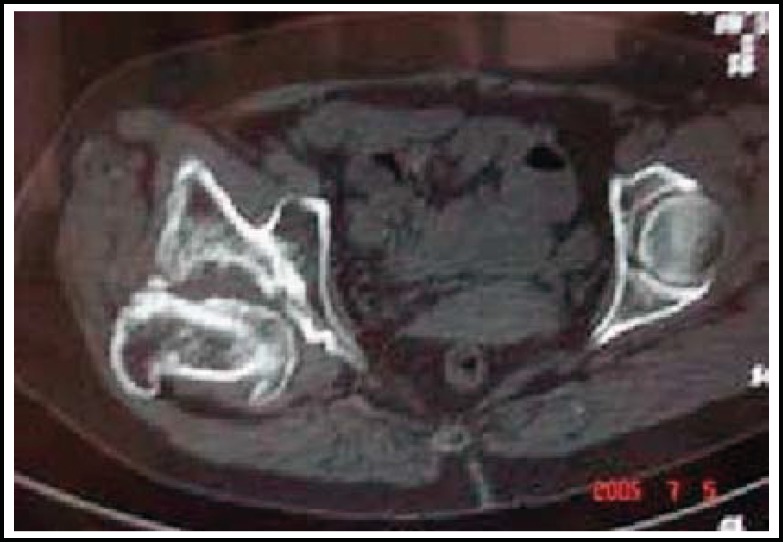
Fig.2 CT scan showed the acetabular defect in posterior column and posterior wall.
